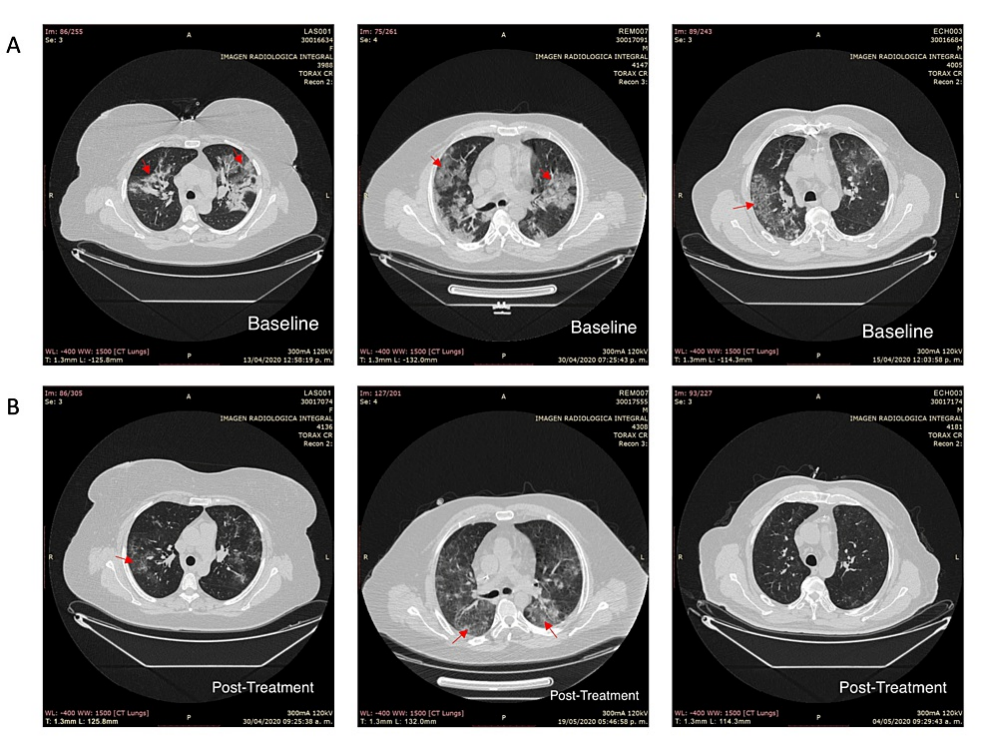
CT scan images of three patients. (A) Baseline chest imaging before MSC treatment. Images from left to right were patient number 001, 007, and 003. (B) Follow-up images after MSC treatment. Images from left to right were patient number 001, 007, and 003. MSC: Mesenchymal stem cells
